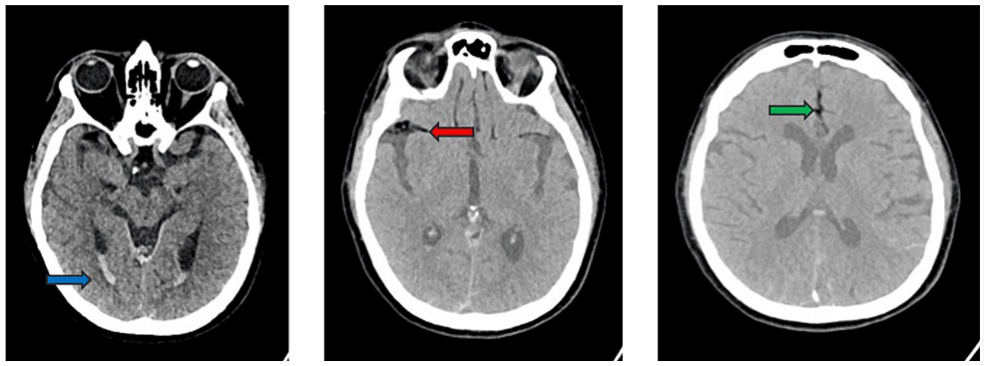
Axial CT images of the brain showing intraventricular bleed (blue arrow), as well as fat density material within the anterior aspect of the right sylvian fissure (red arrow) and within the anterior interhemispheric fissure (green arrow).
